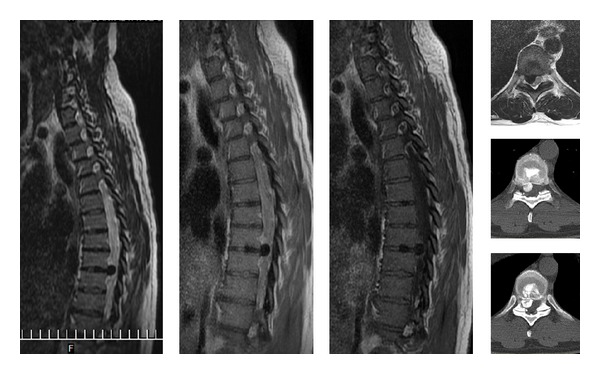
36-year-old female. Weakness in lower extremities. Preoperative ASIA was C (Case 5). Preoperative CT and MRI revealed a thoracic 8-9 disc herniation.
